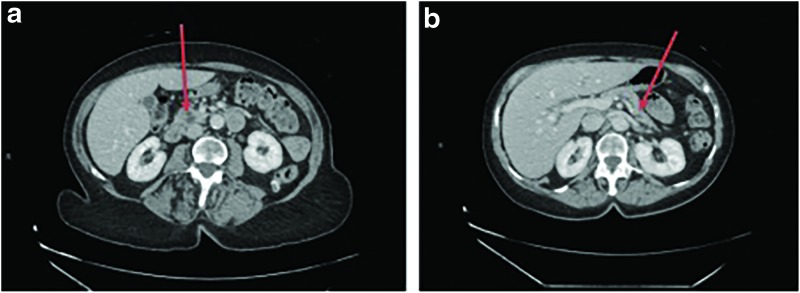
CT scan showing two cystic pancreatic lesions. Left panel (a) shows a 6 mm lesion in uncinate process (arrow). Right panel (b) shows a 5 mm lesion in tail of pancreas (arrow), and a dilated main pancreatic duct in the body and tail. CT, computed tomography.
